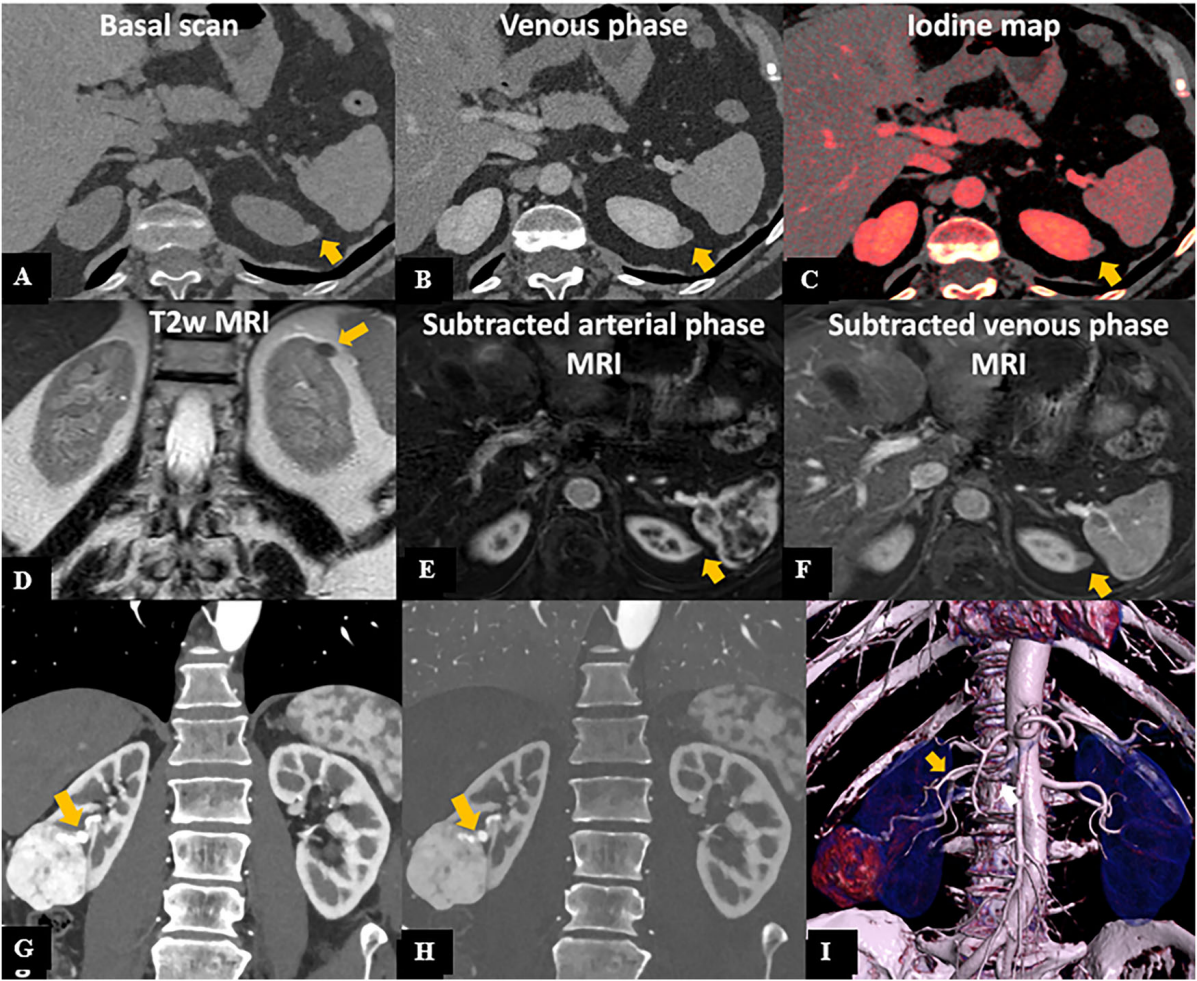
PCCT in renal cancer. A 57-year-old woman undergoing PCCT. Axial pre-contrast (A) and venous phase (B) scans show a small exophytic nodule of the superior pole of the left kidney (11 mm), slightly hyperdense in both phases, with mild iodine uptake at the iodine map (C), suggestive of a small papillary neoplasia rather than a hemorrhagic cyst. MRI confirmed the presence of a small left kidney nodule, hypointense in the T2w image (D), characterized by mild enhancement in the arterial phase (E), with minimal washout in the venous phase (F), thus confirming PCCT suspicion. PCCT in kidney tumor surgery planning: coronal angiographic CT scan showing an exophytic heterogeneous lesion in the mid-lower right kidney (G), 5 cm in diameter, with a well-defined hypertrophic vascular pole, further highlighted on the iodine map (H). 3D reconstruction shows a double right renal artery; the lesion is supplied by the more caudal branch (white arrow in I)
